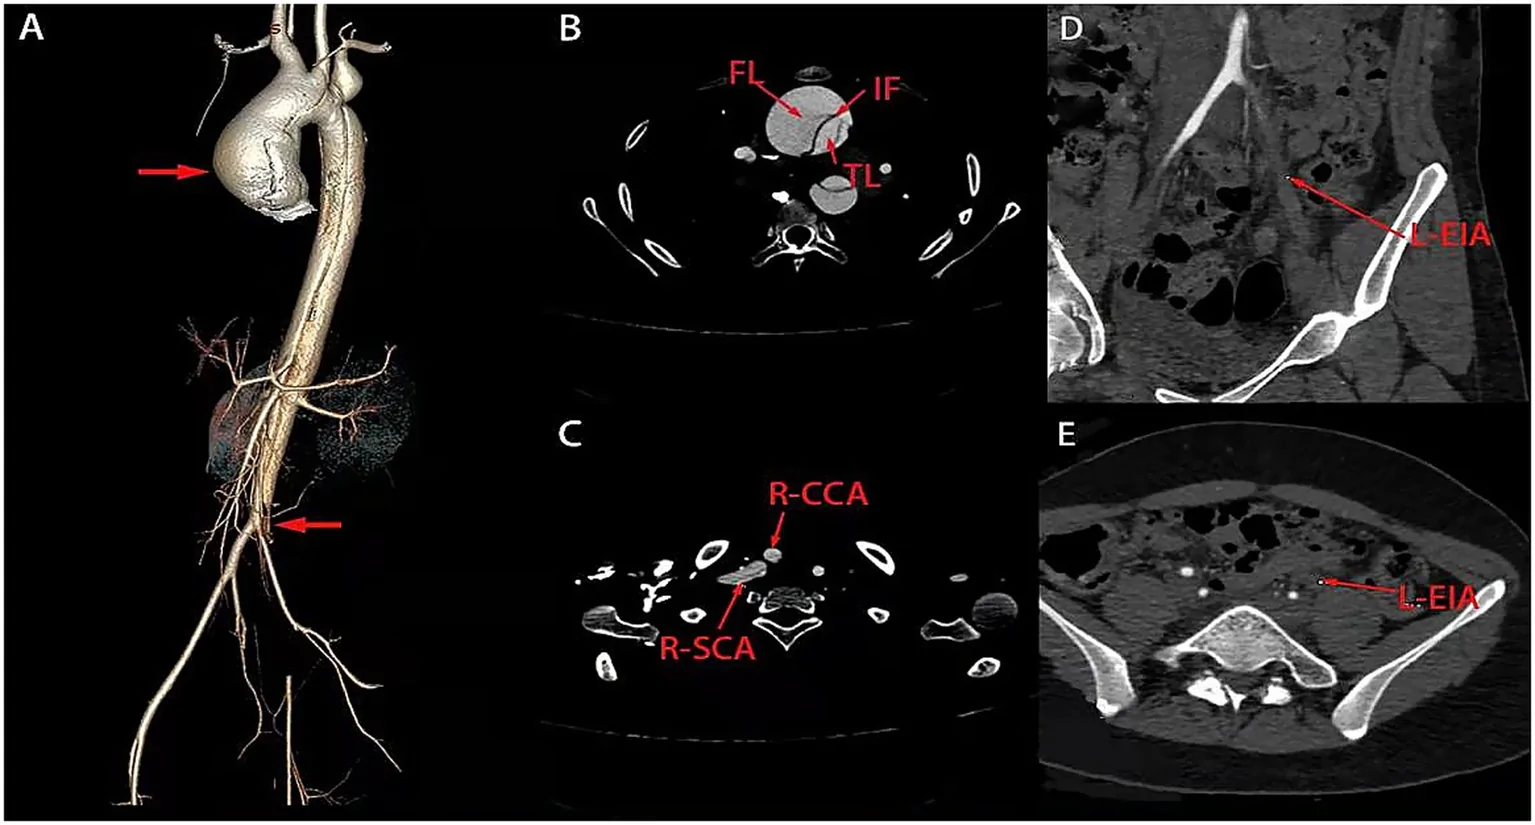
Computerized tomographic angiography (CTA) of the aorta artery (AA): (A,B) demonstrated that the ascending aorta enlarged markedly, with spiral tears throughout the endovascular range, from the root of AA to L-CLA; (C) two-chambered manifestation of R-SCA; (D,E) L-EIA was undeveloped in the CTA. L-CIA, left common iliac artery; L-EIA, left external iliac artery; R-CCA, right common carotid artery; R-SCA, right subclavian artery; TL, true lumen; FL, false lumen; IF, intimal flap.
